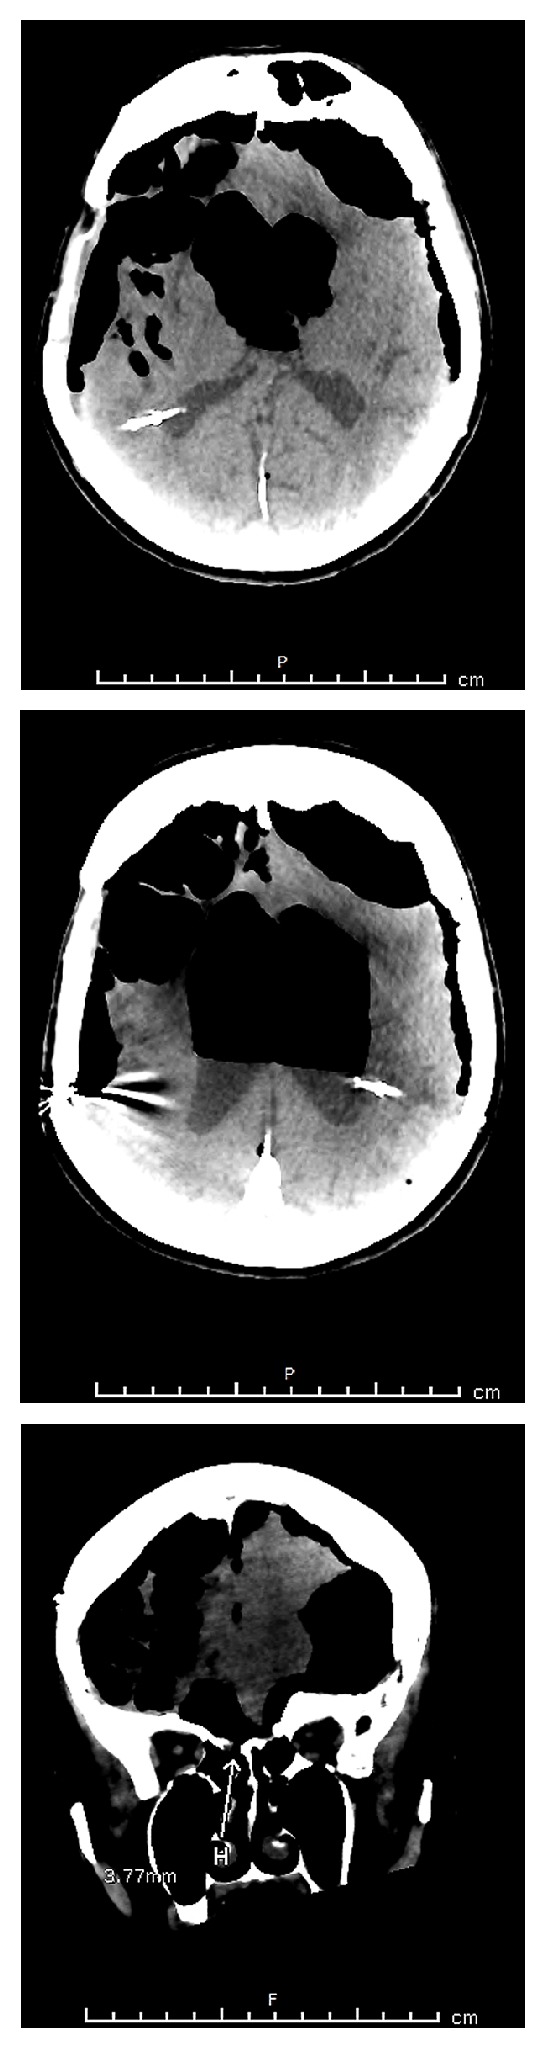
CT-brain scan shows large amount of intracranial free air and bony defect at the right cribriform plate after starting the patient on nasal cannula.
